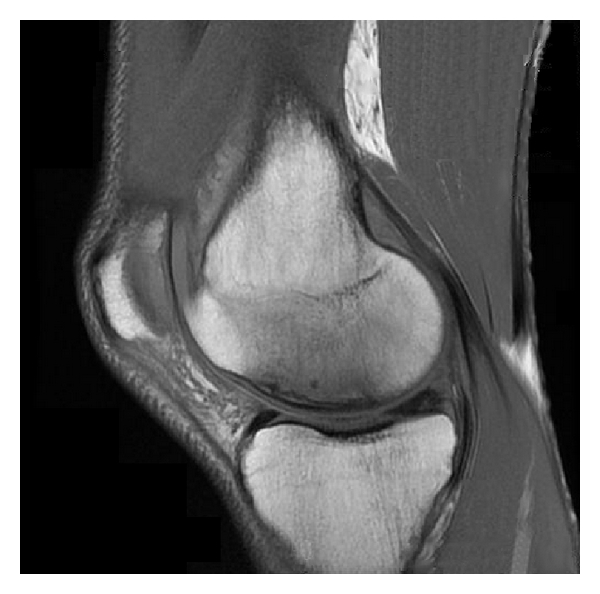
MRI of right knee demonstrating flattening and irregularity of the articular surface with small cyst formation and alteration of the marrow signal, which suggests osteonecrosis.
